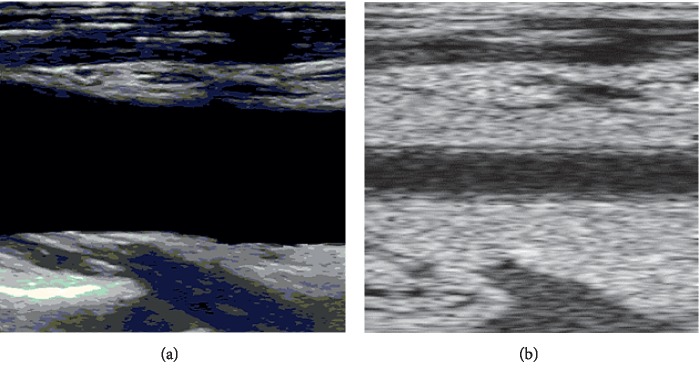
Clinical B-mode ultrasound image after removing the part of the wall (a) and the synthetic image (b).
